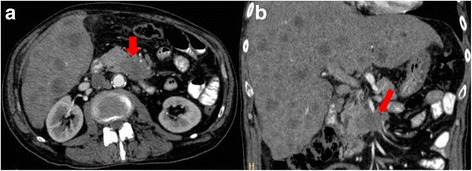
CT scan in axial plane (a) and coronal plane, Multiplanar Reconstruction-MPR (b), during pancreatic phase of dynamic contrast study. Body-tail adenocarcinoma (arrow)
